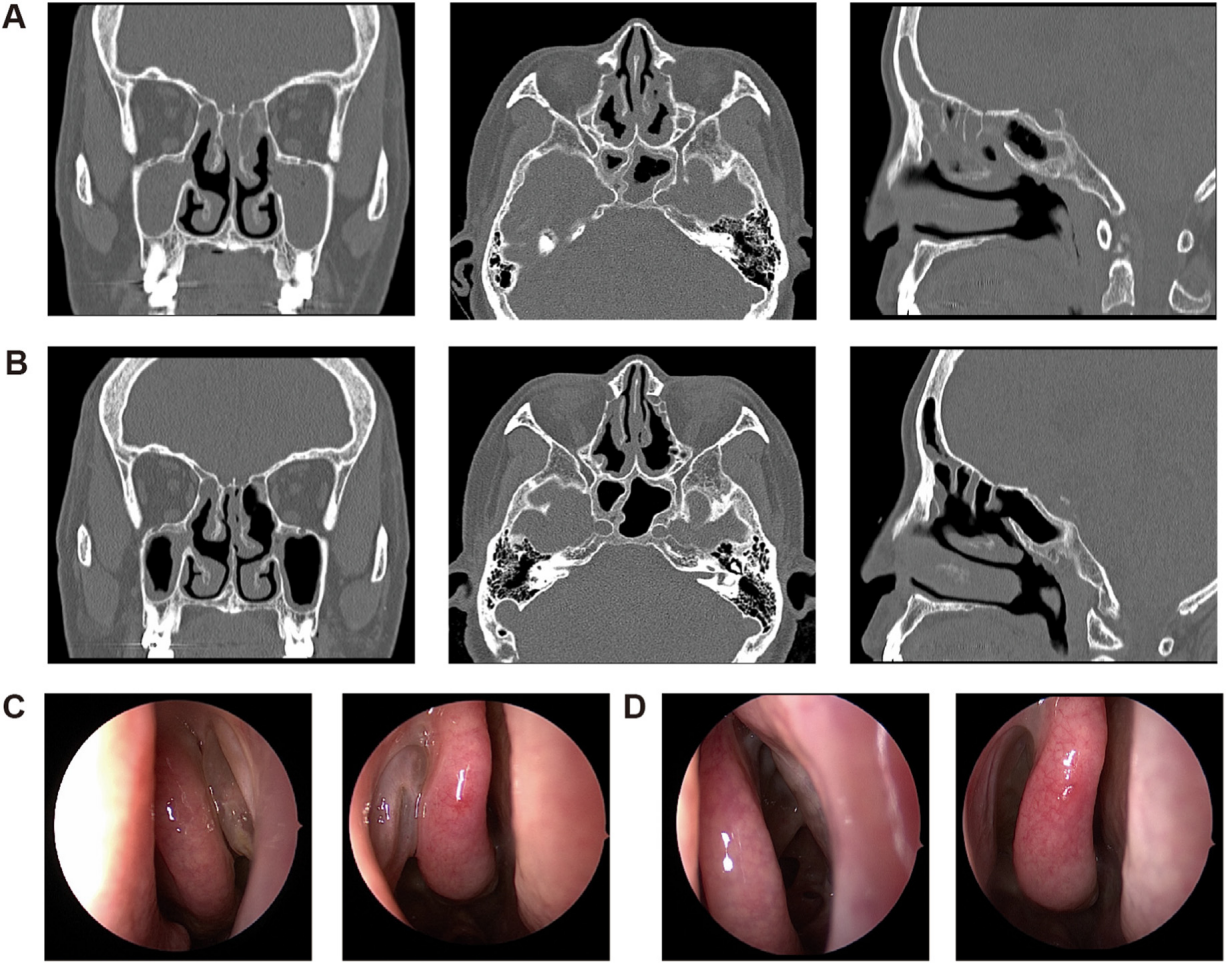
Comparison of objective examination in one patient at week 0 and week 24. A and C were the patients' CT scan and endoscopic pictures at baseline, and B and D were the patients' CT scan and endoscopic pictures after 24-week treatment with omalizumab.
